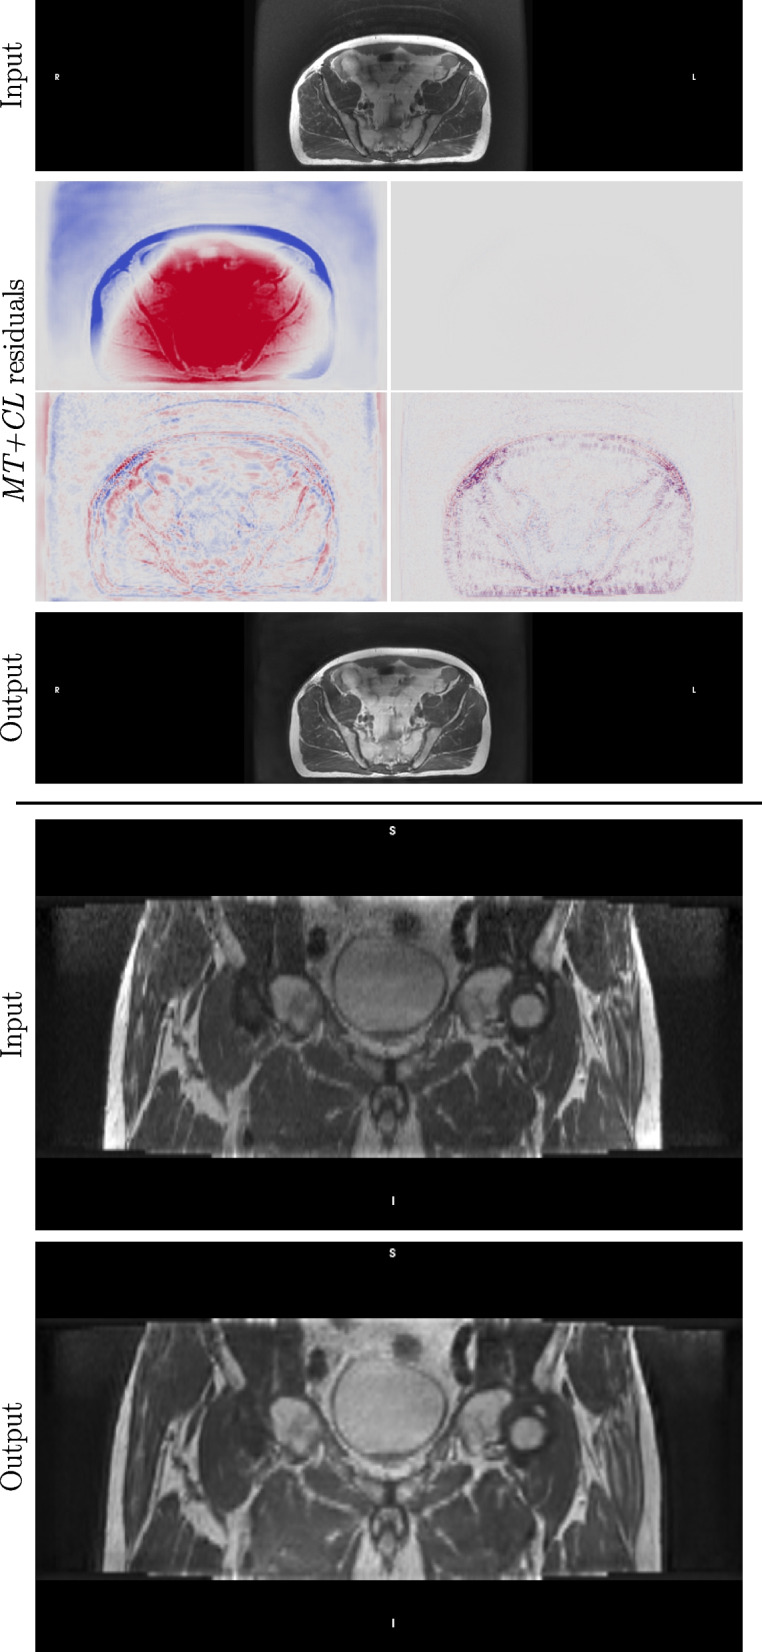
Example corrections using the model *Pelvis MT+*$$\mathcal {L}_C$$ for pelvic MRI scans from Gold Atlas of two patients. The top part shows a slice from the first patient with the input slice (top), the prediction (bottom) and the output residuals for bias, subsampling, motion and noise, respectively. The bottom part shows another scan of 77 axial slices from the coronal view (top) and the corresponding corrections by the model (bottom)
